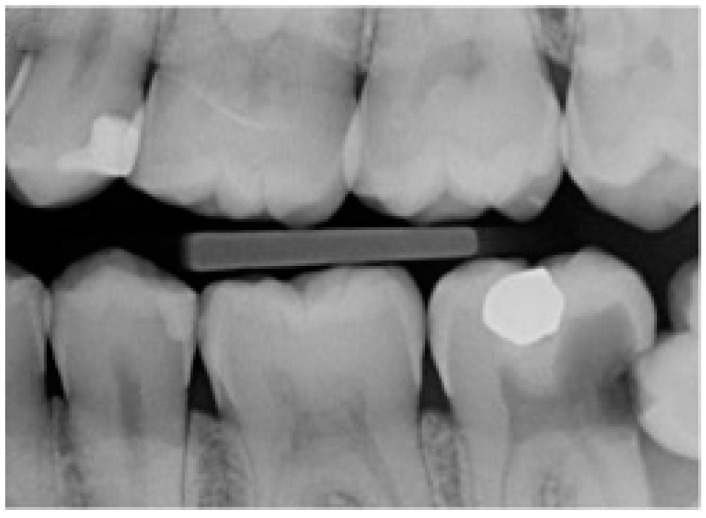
Left bitewing radiograph of a partially erupted and impacted mandibular third molar.
